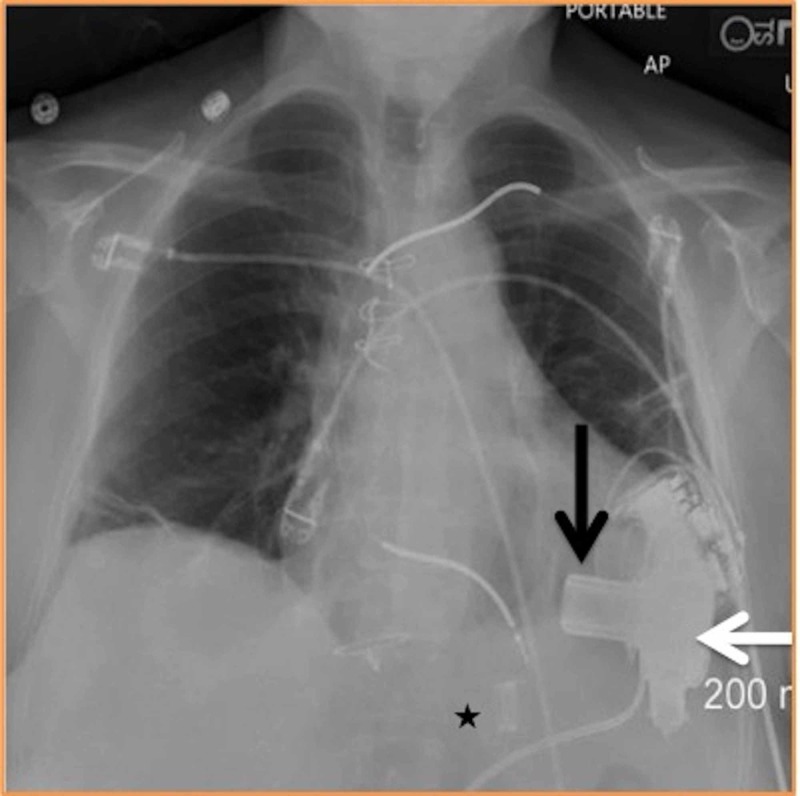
Plain radiograph “HVAD (HeartWare)” 3rd generation left ventricular assist device (LVAD). Black arrow – inflow cannula; white arrow – pump; black star – outflow cannula.
